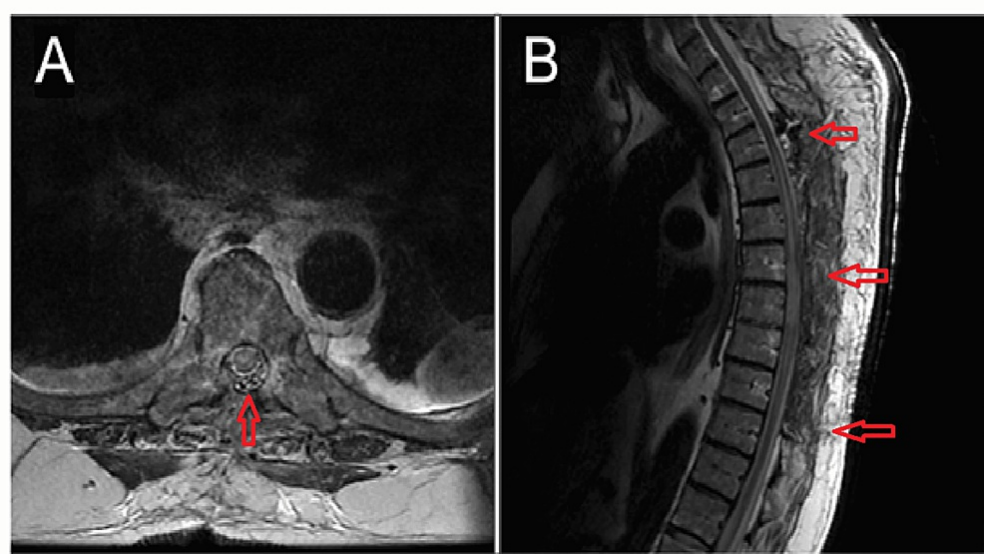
Postoperative T2-weighted MRI demonstrating post-surgical decompression and absence of thecal sac displacement. A) axial view, B) sagittal view
